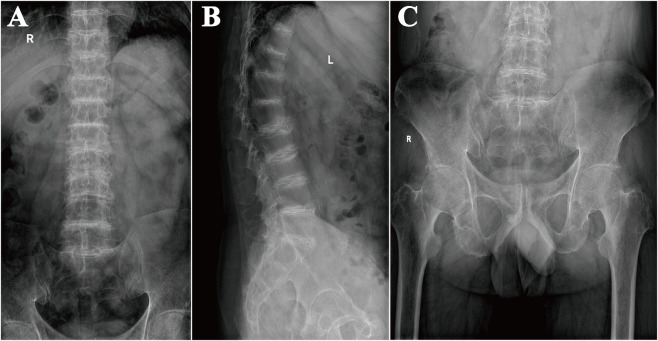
Radiographic findings of the lumbar spine and pelvis. (A,B) Lumbar spine radiographs demonstrating degenerative changes, including decreased bone density, loss of lumbar lordosis with sagittal imbalance, multilevel endplate sclerosis from T9 to S1, and intervertebral disc spaces narrowing with calcification. (C) Pelvic radiograph showing degenerative changes with symmetrical cortical irregularities and osteophyte formation at the bilateral iliac crests, greater trochanters, and ischial tuberosities.
